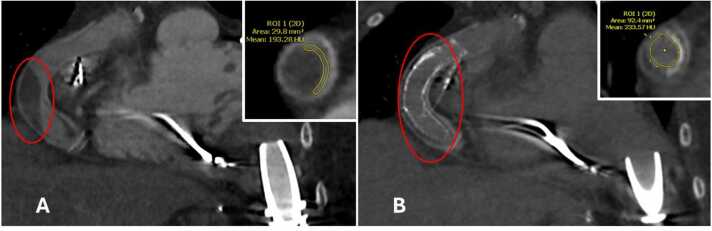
CT chest pre- and post-stenting. (A) CT chest pre-stenting. Area of minimal flow of 29.8 mm^2^. LVAD flow of 2.5 liter/min. (B) CT chest post-stenting. Area of minimal flow of 92.4 mm^2^. LVAD flow of 5 liter/min. CT, computed tomography; LVAD, left ventricular assist device.
